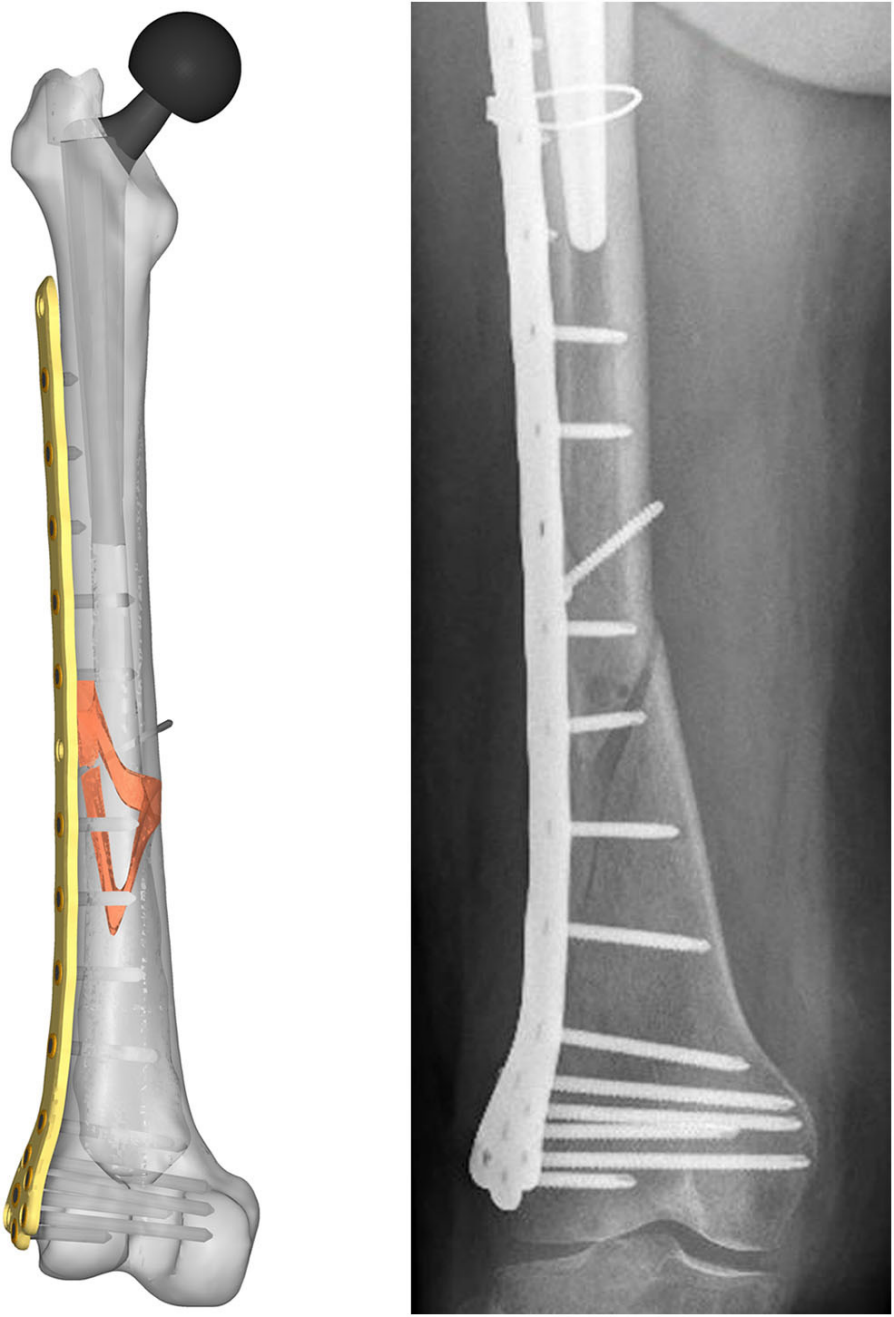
Resulting assembled geometrical model (Left) compared with the corresponding x-ray image (Right).
